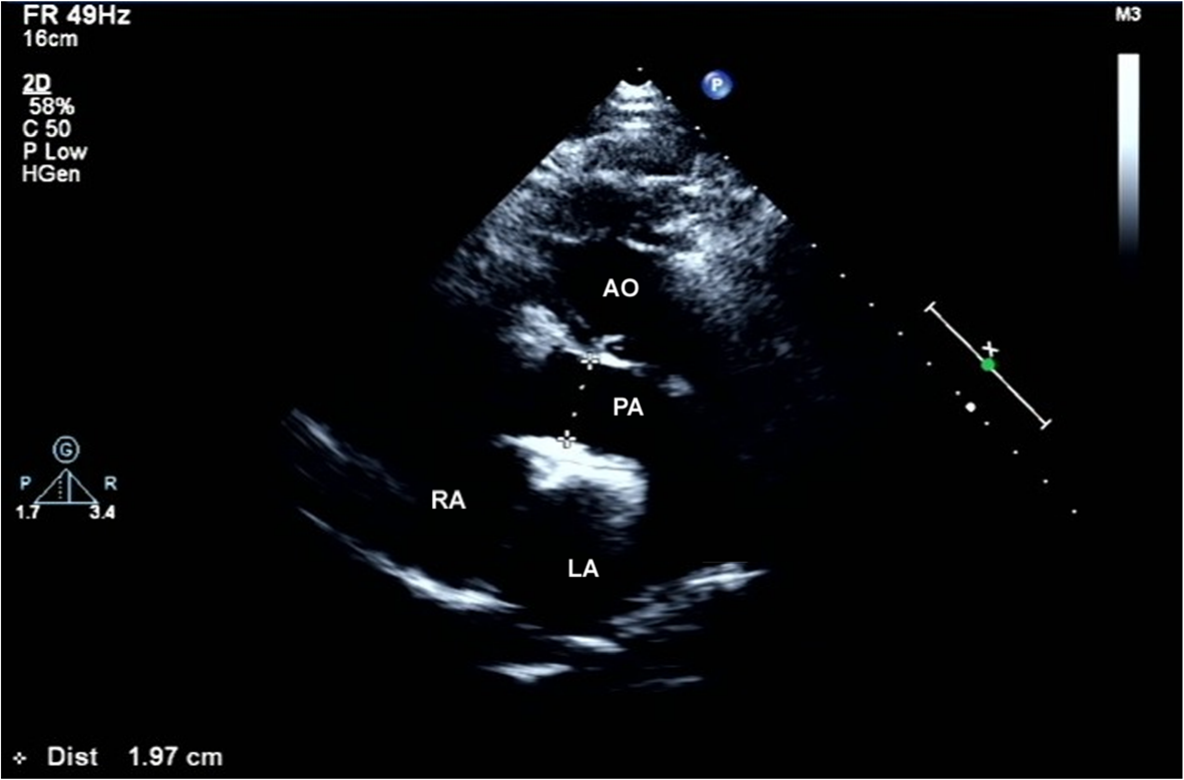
Two dimensionally parasternal long-axis view showing the aorta lied in anterior left side of pulmonary artery and connected to morphological right ventricle (functional left ventricle). AO = aorta; PA = pulmonary artery; LA = left atrial; RA = right atrial
